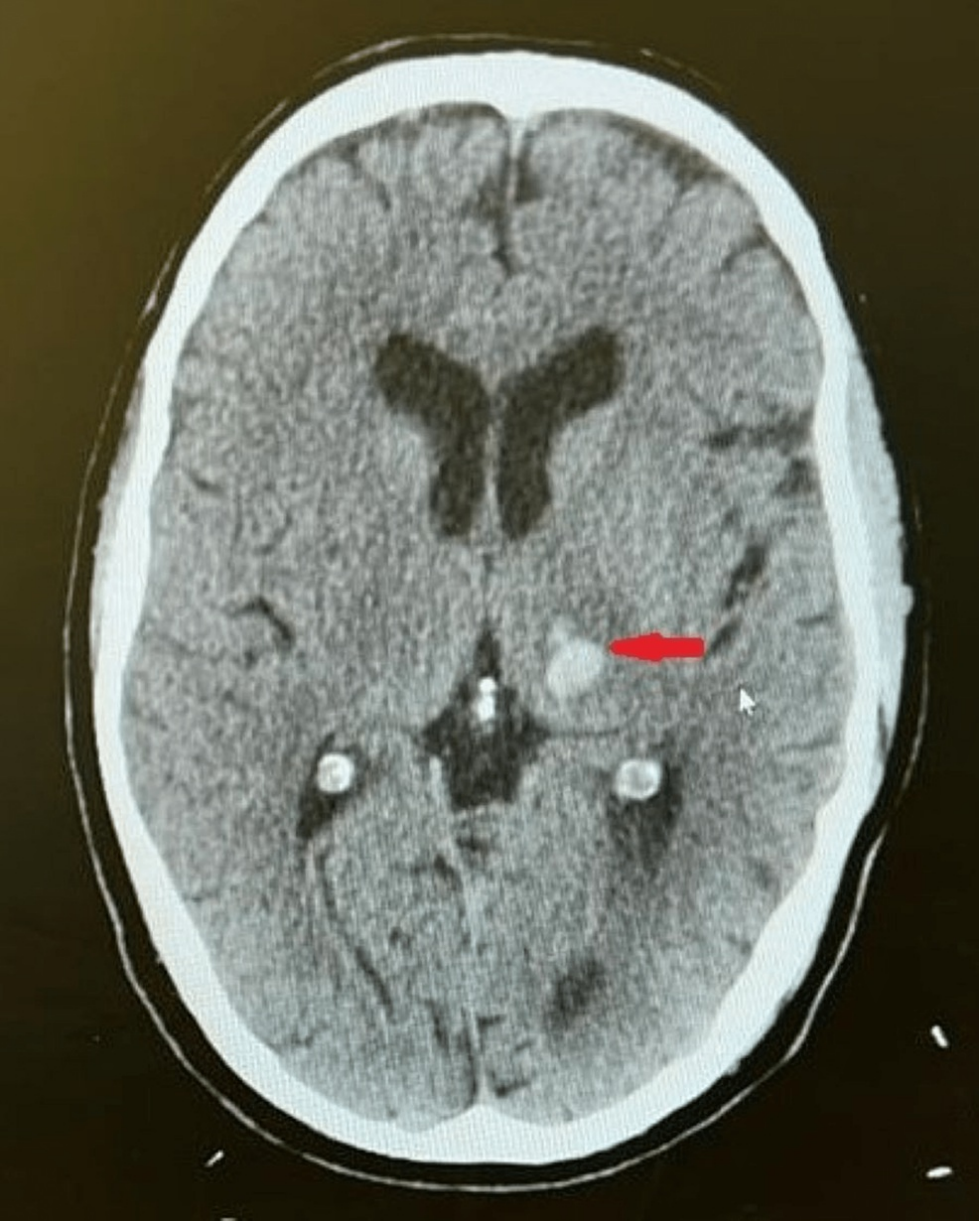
Axial image of a non-contrast CT of the brain showing hyperdense left-sided intracranial hemorrhage (red arrowhead) measuring 10 x 12 mm with surrounding small volume edema. There is no mass effect and no associated midline shift.
